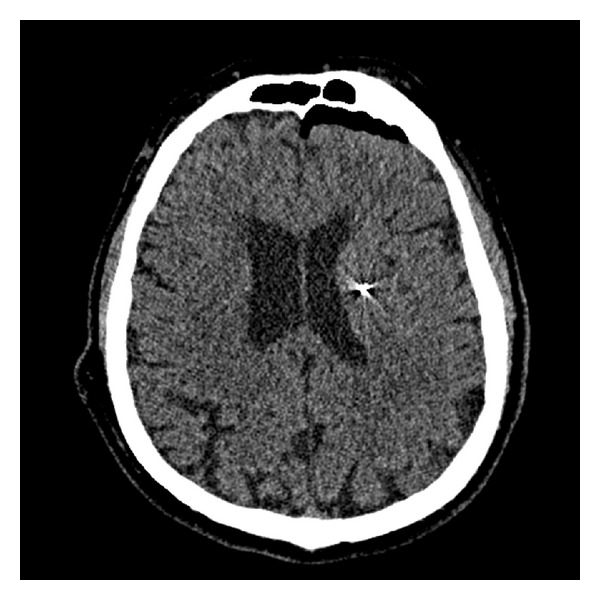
Axial CT without contrast enhancement showing no acute hemorrhage along DBS tract immediately after DBS placement.
